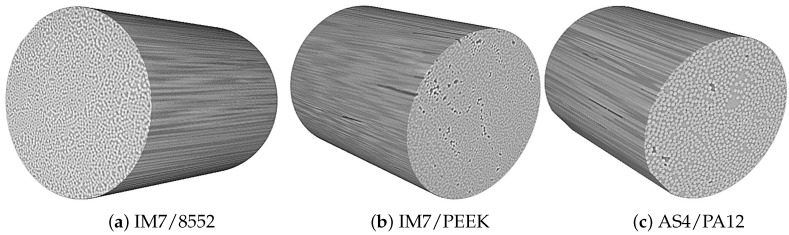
Samples of the constructed computed tomography (CT) scans for the entire test campaign.
